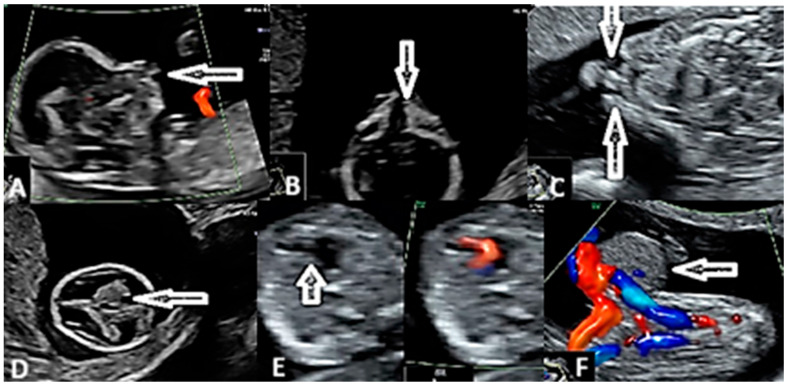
(A) Abnormal fetal profile. (B) Fetal face—cleft palate (arrow). (C) Fetal face—bilateral cleft upper lip (arrows). (D) Abnormal fetal brain. (E) Fetal heart—hypoplastic left heart (arrows; Left side-Grey Scale and Right side-Color Doppler Mode) (F) Fetal abdomen—omphalocele (arrows; Grey Scale and Color Doppler Mode).
